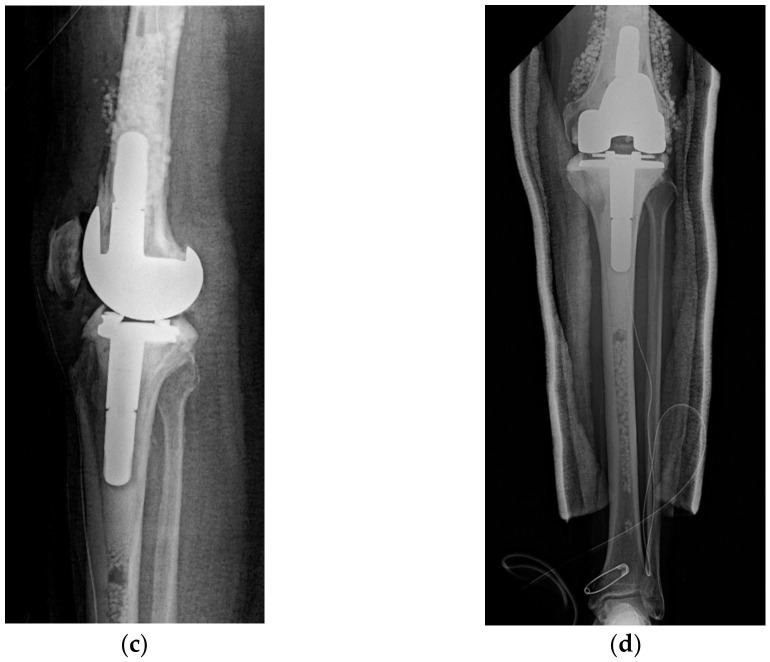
(a–d): Radiographs demonstrating articulating 1.5 exchange TKA. (a) Anterior–posterior (AP) view of proximal femur showing ALCSB delivered within the medullary canal using 3.0 and 4.6 mm beads. In this case 40 cc CaSO_4_ powder (≈100 cc bead volume) was delivered within the medullary canals and joint. (b) AP knee showing constrained revision knee implants cemented with high-dose antimicrobial loaded cement. Stems are short and smooth. Note that tibial implant is one size smaller than trial size to circumferentially coat the polyethylene insert. The periphery of the polyethylene is drilled with a 5.0 mm drill to allow the cement to interdigitate. Also note antimicrobial loaded CaSO_4_ beads filling medullary canals and compacted serving as cement restrictors. (c) Lateral knee view showing the patella resurfaced with an undersized implant with cement completely covering the patellar bone and polyethylene except for the apical 33% of the polyethylene dome. Note again how beads serve as a cement restrictor. (d) AP tibia showing ALCSB delivered within the medullary canal using 3.0 and 4.6 mm beads.
